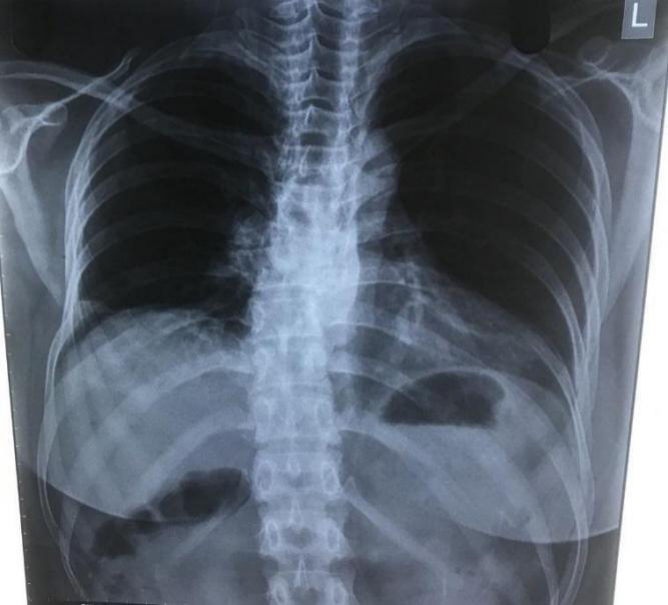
Chest X-ray posteroanterior (PA) view showing deformity at T8-T9 level along with the posterior aspect of the 4th and 5th ribs fracture near costovertebral junction.
